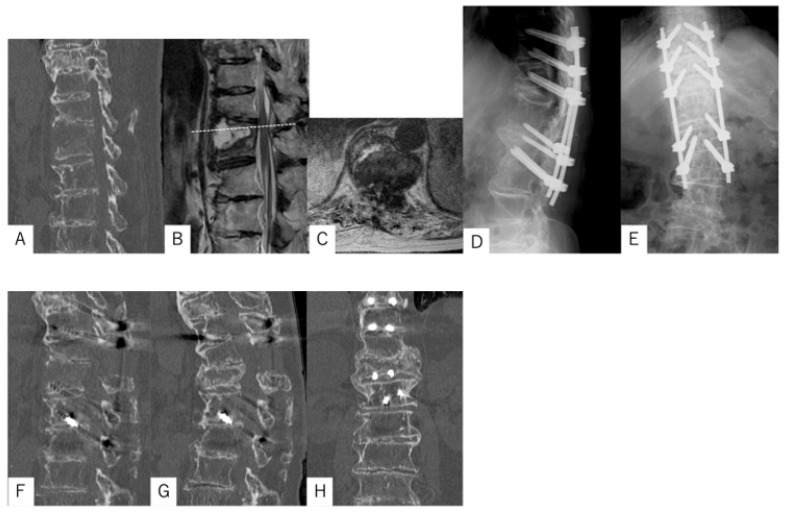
Spinal fracture case with DISH involving an 89-year-old woman. (A) Initial CT image showing a marked anterior distraction gap (19 mm) at Th12. (B) Initial sagittal magnetic resonance imaging (MRI) image. (C) Initial axial MRI at the level indicated by the dotted line in panel (B), showing spinal canal stenosis at the Th11/12 level. (D) Postoperative posteroanterior radiogram. (E) Postoperative lateral radiogram. Posterior fixation was performed from Th9 to L2 (five levels) using TSDs, with a laminectomy from Th11 to Th12. (F) CT image 1 week postoperatively showing a residual anterior gap of 14 mm. (G) Sagittal CT 12 months postoperatively showing complete closure of the gap (0 mm). (H) Coronal CT 12 months postoperatively demonstrating sufficient bone bridging and union with no implant loosening.
